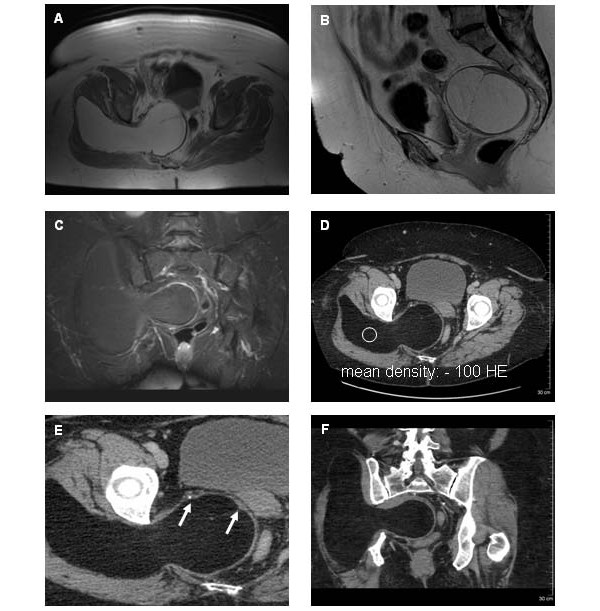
Pelvic MR and CT imaging. A) Massive fatty tumor with hyperintense appearance in the noncontrast T1-weighted (TR/TE, 168/5) axial image. B) The sagittal noncontrast T1-weighted (TR/TE, 600/10) image demonstrated a thin septum inside the tumor. C) The tumor showed a complete signal loss in the fat saturation sequences (TR/TE 5660/48). D) Axial noncontrast multidetector CT image demonstrated a massive soft-tissue pelvic tumor occupying the sciatic foramen. The mean density was -100HE and identified the fatty tissue characteristics. E) Magnification of the axial noncontrast multidetector CT image revealed nodular appearance of the septa (see arrows). F) Coronal reconstructed noncontrast multidetector CT image showed the expansive soft-tissue mass inside and outside the pelvic. Adjacent organs und muscles were displaced.
